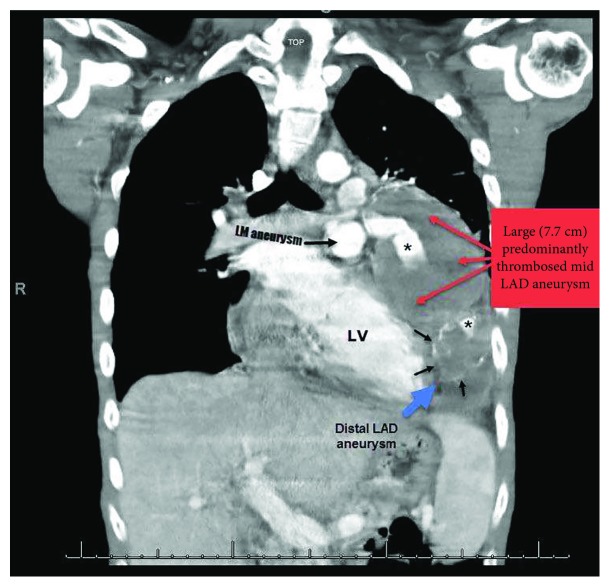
Computed tomographic scan shows a large mostly thrombosed proximal and mid left anterior descending artery (LAD) aneurysm (red arrows) measuring 7.7 cm in addition to a smaller calcified distal aneurysm (blue arrow). The opacified left main (LM) aneurysm is also seen.
